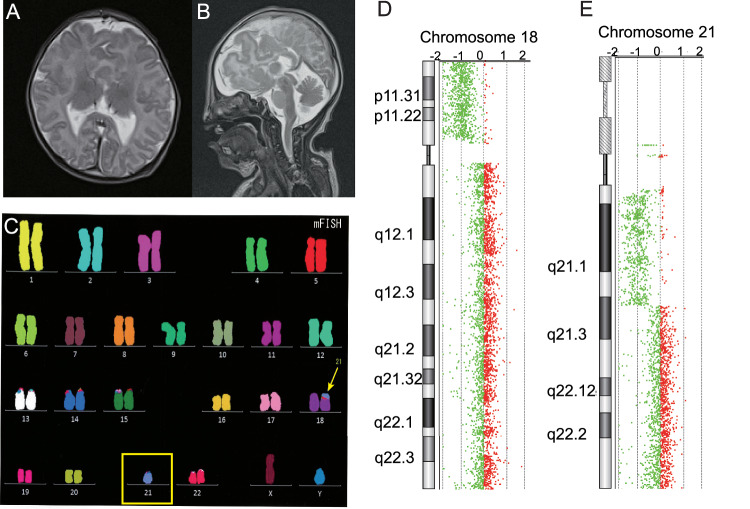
Clinical and genetic findings of the patient. A, B Brain magnetic resonance imaging at 8 days of age. T2-weighted axial A and sagittal B images show lobar HPE. C Multicolor FISH of the patient. An unbalanced translocation at 45,XY,der(18)t(18;21),-21 was revealed. The yellow box shows -21, and the arrow indicates der(18)t(18;21). D, E Array CGH of the patient. The vertical axis represents the chromosomal position, while the horizontal axis shows the fold change in copy number variation. Array CGH revealed 45,XY,der(18)t(18;21)(p11.2;q21.3),-21, 13.8-Mb deletion at arr[GRCh37] 18p11.32p11.21(131,700_13,935,908)×1 D and 12.7-Mb deletion at arr[GRCh37] 21q11.2q21.3(14,957,733_27,609,147)×1 E.
